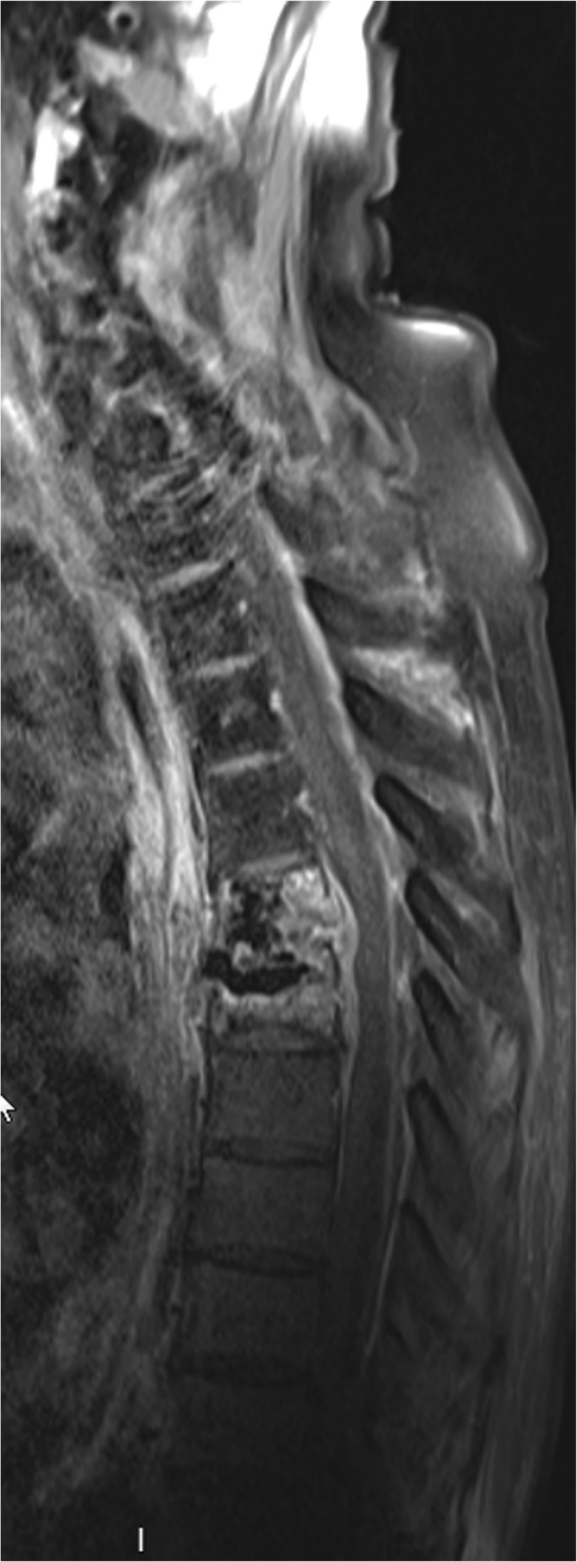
Spine MRI shows improvement from earlier imaging, with decrease of epidural abcess and of medullary compression. Resolution of the abscess surrounding the site of cementoplasty as well as anterior abscess at the level of third thoracic to six thoracic and the soft tissue abcess
